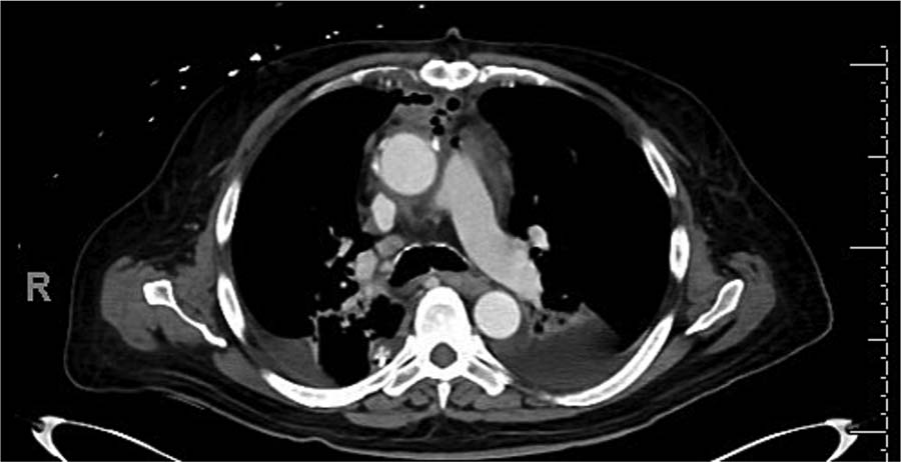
Post operative CT scan concerning for mediastinitis.
